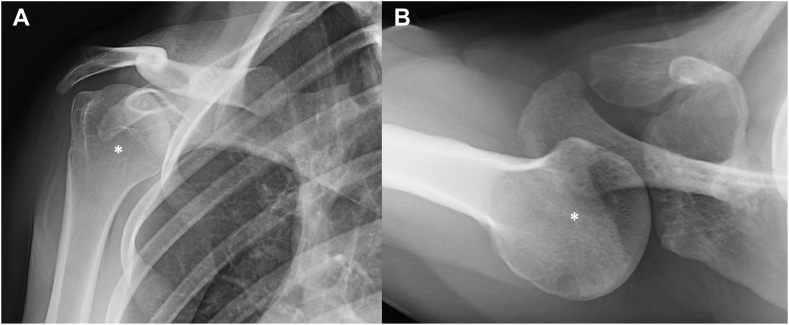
Grashey (A) and axial (B) radiographs of the contralateral right shoulder at 24 months of follow-up. Both views demonstrate a concerning posterior subluxation of the shoulder with glenoid retroversion, similar to the initial presentation of the left side.
